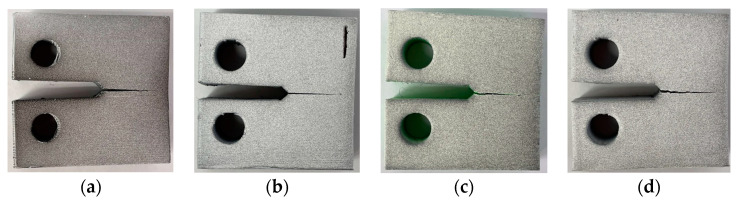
Fractured CT samples (Type D) made of various materials: (a) ABS, (b) ABS + CF, (c) ABS + GF, and (d) ABS + BF.
